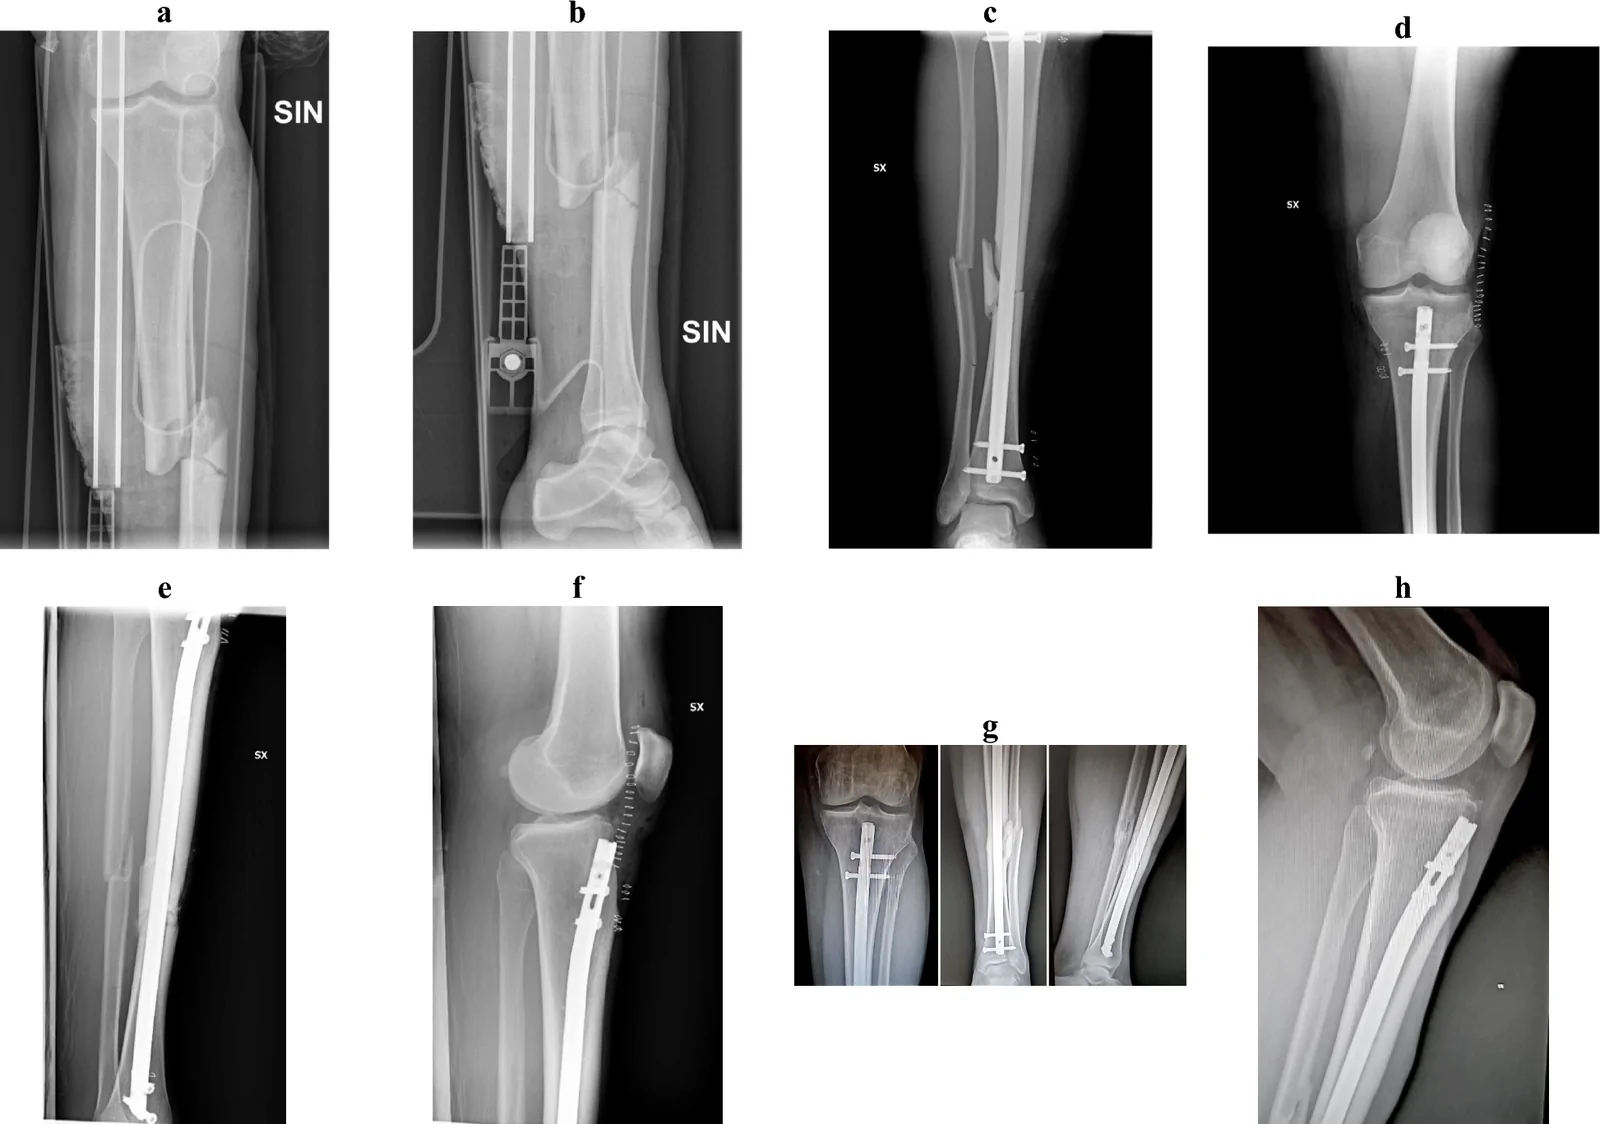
Representative case of a 27-year-old man with an AO/OTA 42-A2 tibial shaft fracture treated with a Bactiguard-coated intramedullary nail. (a, b) Preoperative anteroposterior and lateral radiographs. (c–f) Immediate postoperative radiographs following definitive fixation with a Bactiguard-coated intramedullary nail. (g, h) Radiographs obtained at the 20-month follow-up, demonstrating complete fracture union, restoration of alignment and stable implant fixation
